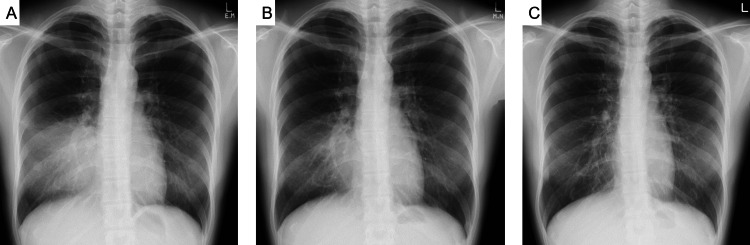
Chest X-ray images during follow-up. A. Chest X-ray on admission showed consolidation in the right lower lung field. B. Eleven days after bronchoscopy, the consolidation showed improvement. C. The shadow had disappeared 53 days after bronchoscopy.
